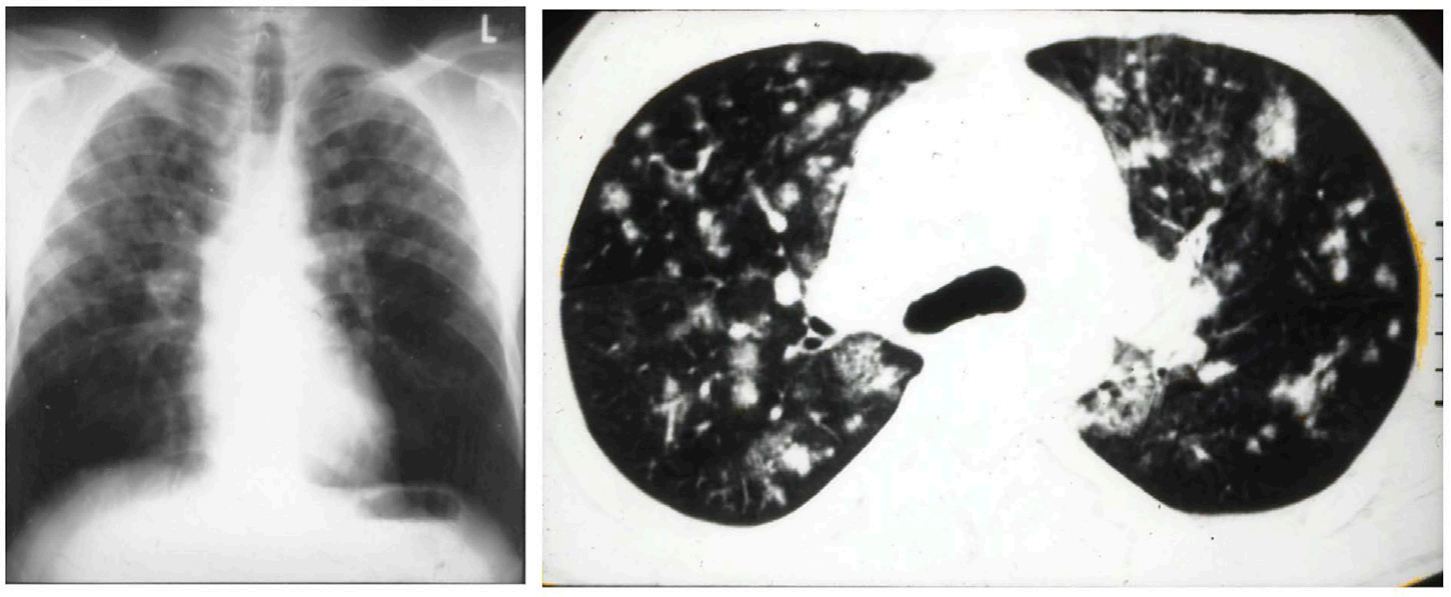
Figure 1 (A) radiograph of a 37-years-old male mountaineer with high-altitude pulmonary edema (HAPE) that shows a patchy to confluent distribution of edema, predominantly on the right side. (B) computerized tomography scan of 27-year-old mountaineer with recurrent HAPE showing patchy distribution of edema. Taken from Bärtsch, P, Mairbäurl, H., Maggiorini, M., Swenson, E. 2005. Physiological aspects of high-altitude pulmonary edema. J Appl Physiol, 98, 1101–1110.
